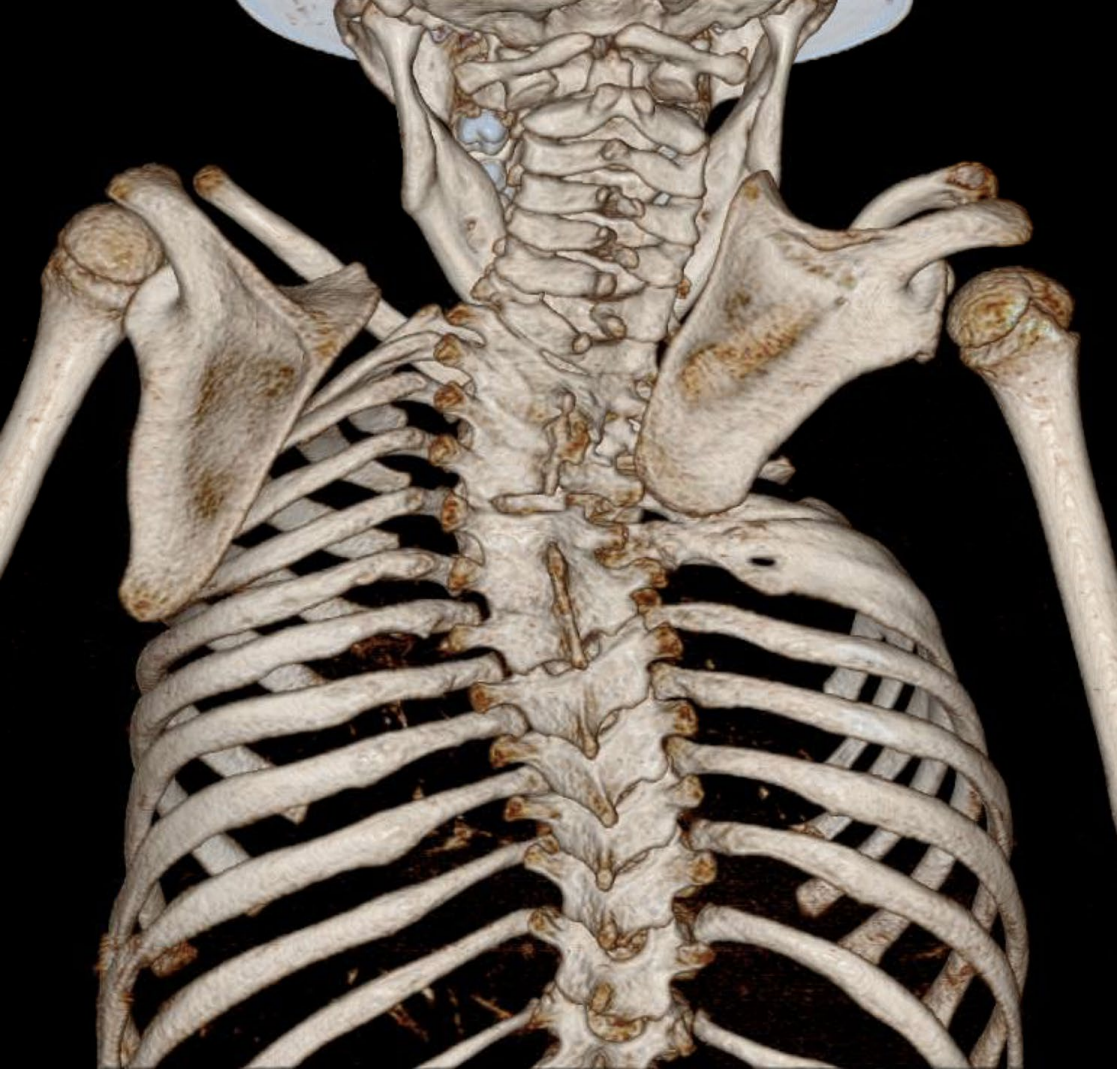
3D-CT scan of a child with unilateral Sprengel deformity of the right shoulder. Preoperative imaging showing Rigault score 3 and associated congenital scoliosis and rib anomalies on the right side
